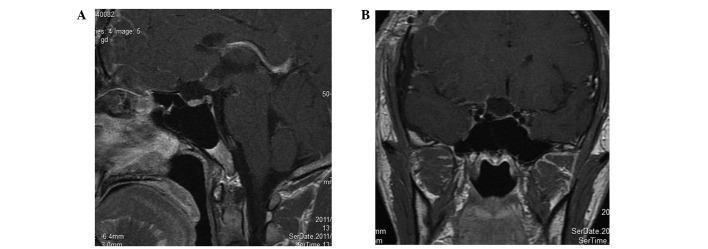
One-week post-operative enhanced magnetic resonance imaging (MRI) demonstrating no tumor recurrence. (A) The tumor shadow has disappeared on the sagittal view. The pituitary and stalk are well preserved. (B) The tumor shadow has disappeared on the coronal view. The pituitary is well preserved.
